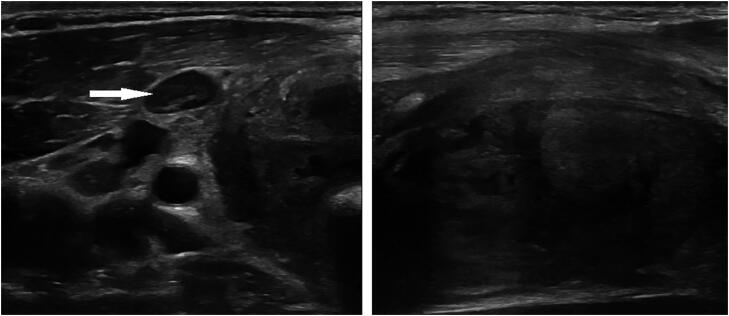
Sonography of the right thyroid lobe at initial presentation (left image: transverse axis, right image: sagittal axis, arrow: prominent lymph node). Images in this figure were previously published in (33) under the Creative Commons CC BY license.
